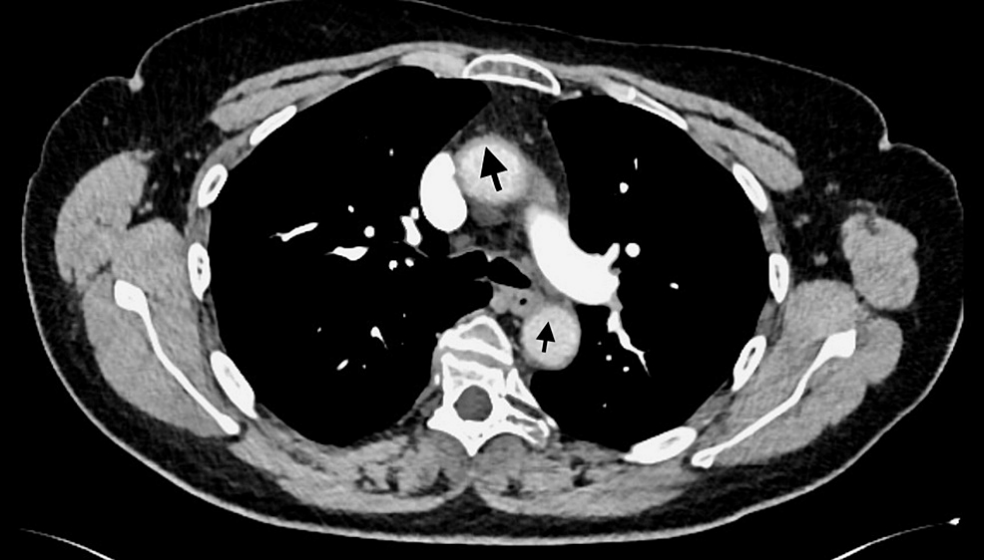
CT scan exhibiting subtle diffuse wall thickening of the ascending and descending aortas (black arrows)
